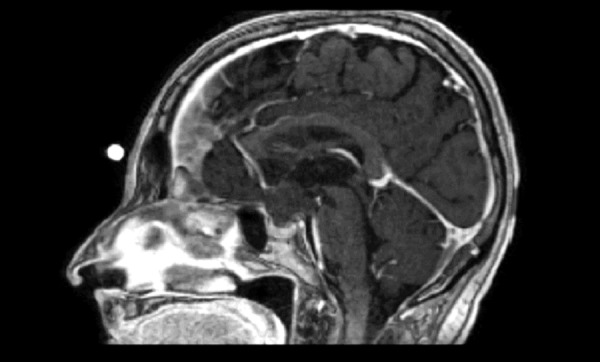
Preoperative sagittal T1 MRI with contrast shows a suprasellar solid-cystic mass compressing the optic chiasm
